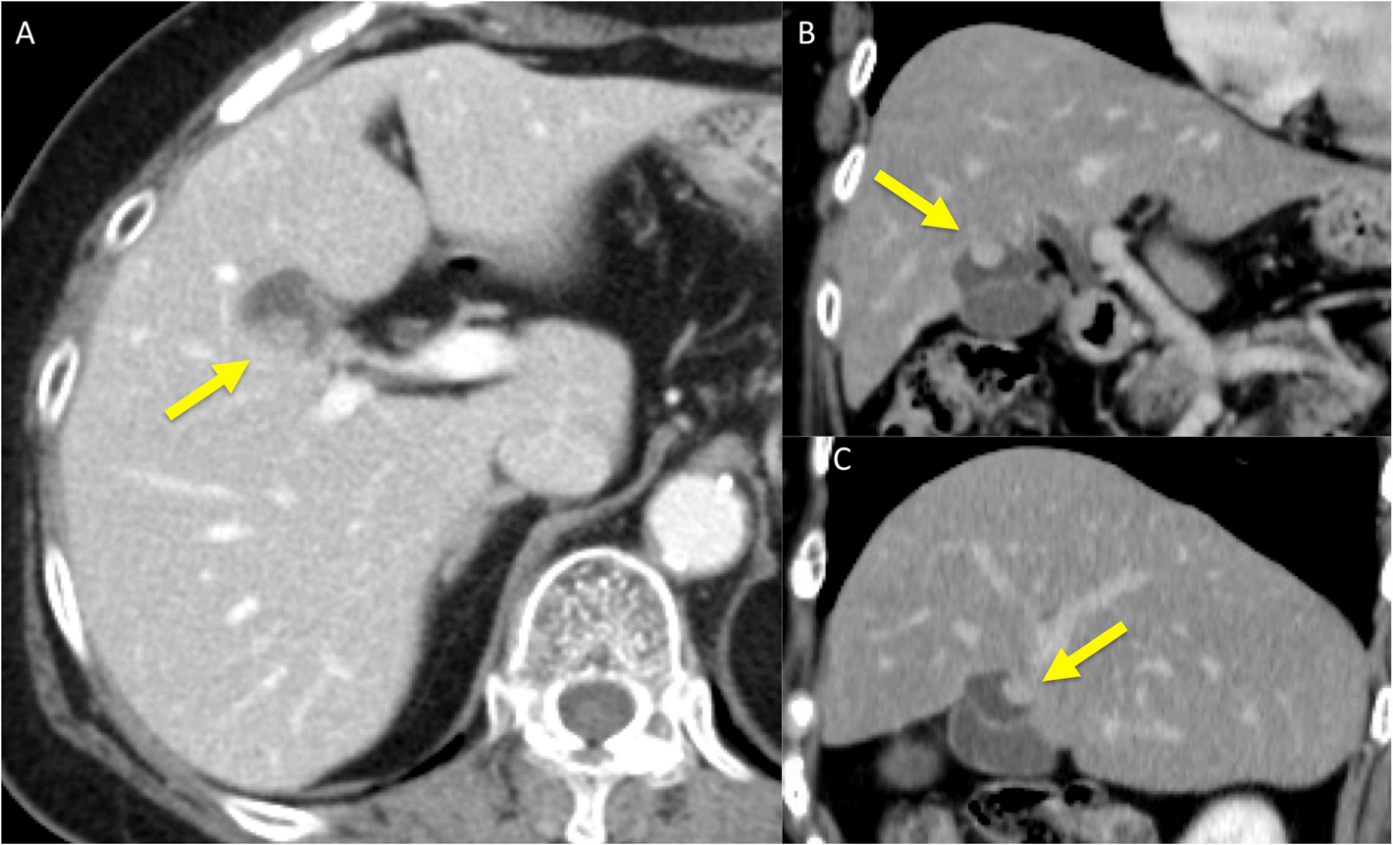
Abdominal enhanced CT imaging. Carcinoma in the proximal gallbladder, which was identified as the accessory gallbladder, shown by enhanced CT images. a Axial view. The low-density mass with a diameter of 1 cm was slightly enhanced (see the arrows). Direct liver invasion was less conspicuous on CT imaging. b Coronal view, and c sagittal view. Two separate cavities were clearly demonstrated
